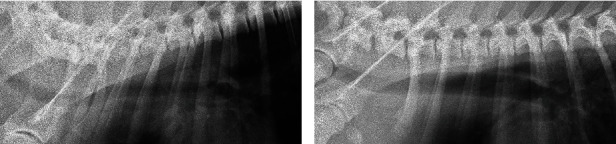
Right lateral radiographs of the thoracic trachea before surgery (a) and after the suction of 100 mL of tracheal fluid (b) in case 1.
